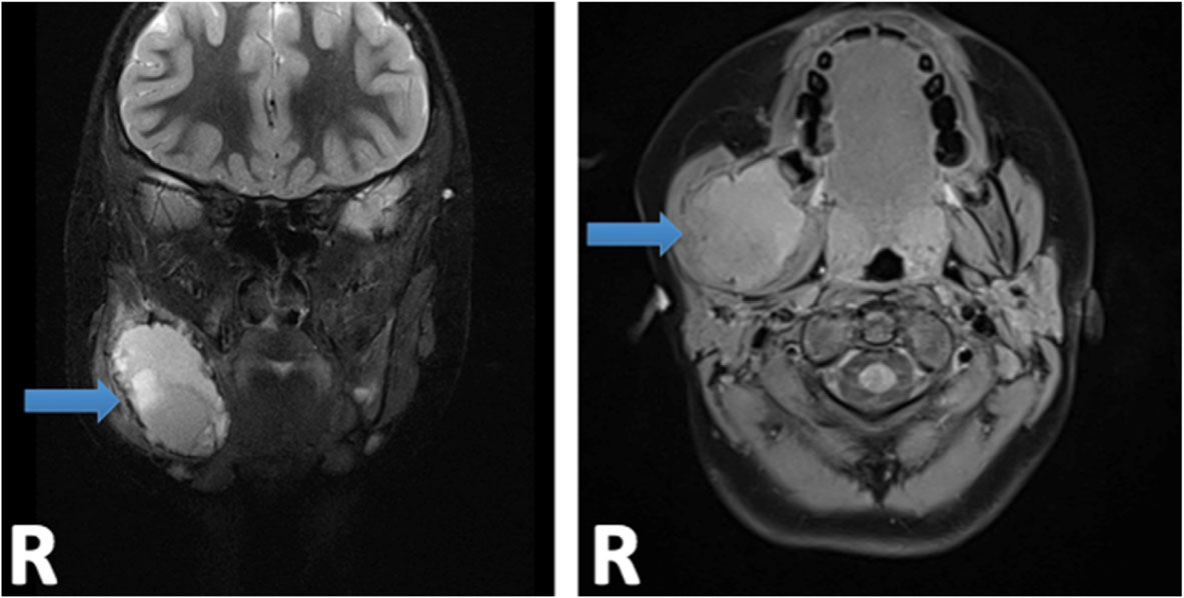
MRI Head following initial presentation to the emergency department with coronal and axial views. Arrows are pointing to site of lesion
